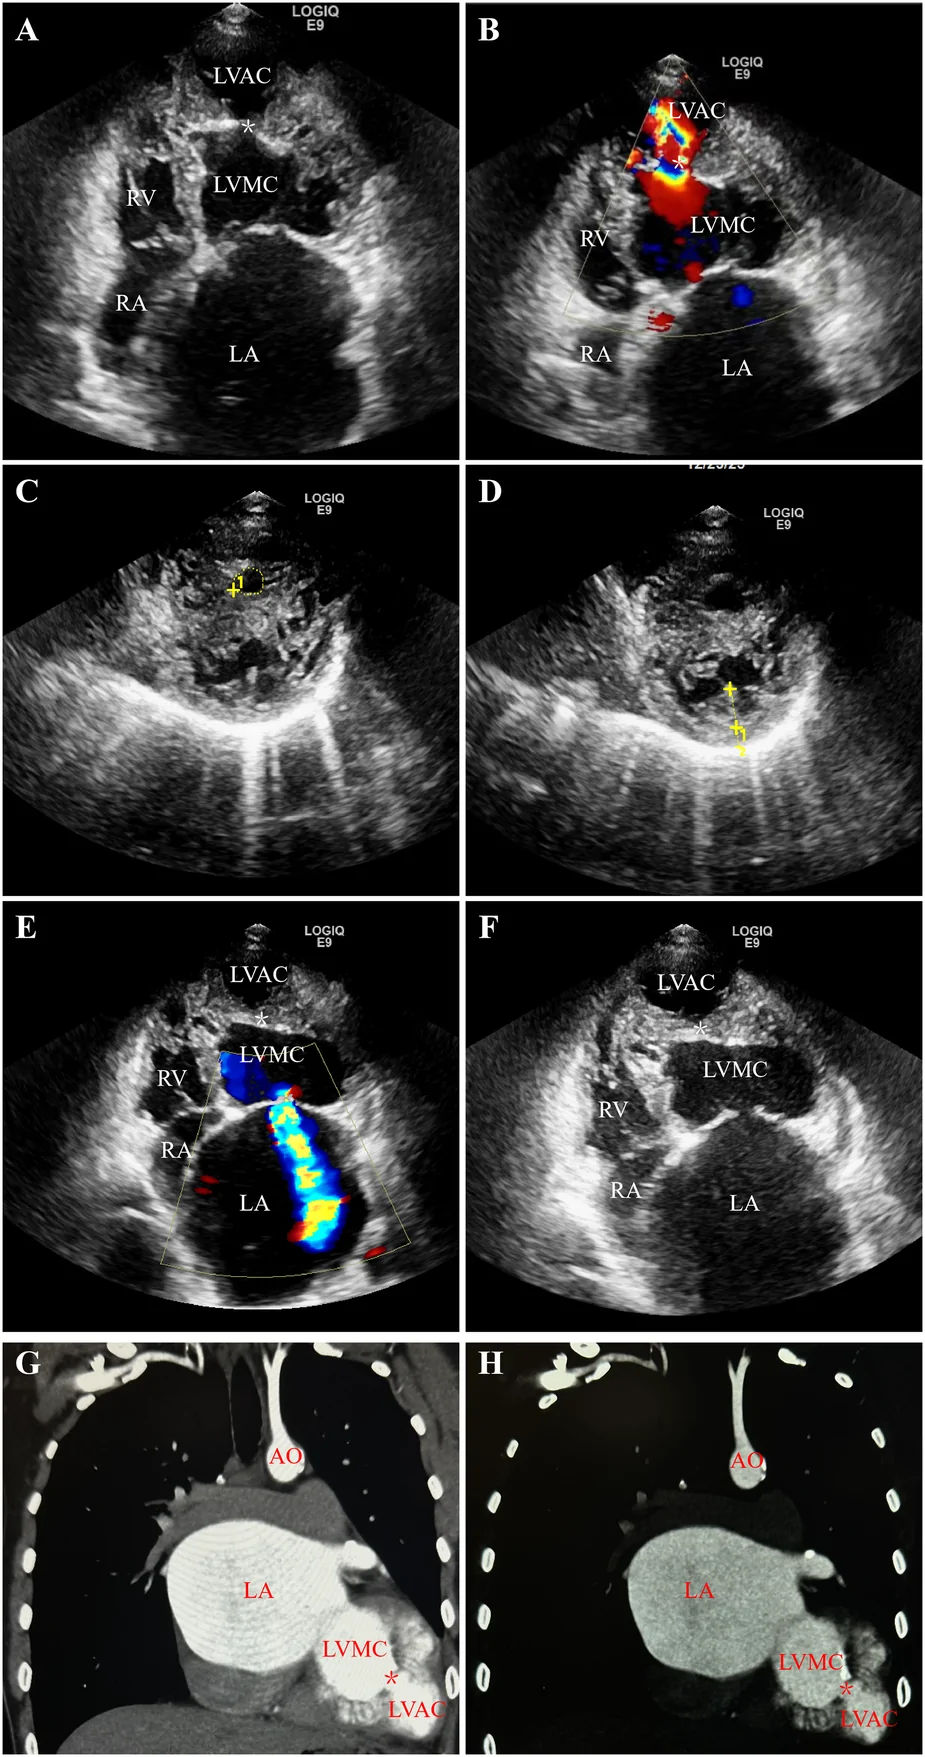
A 50-year-old male with one month of substernal chest tightness and progressive shortness of breath. (A) Echocardiography (Apical four-chamber view): Enlarged left heart is observed. The mid-to-lower segments of the left ventricular wall show thickening and calcification, dividing the left ventricular cavity into two chambers - one near the apex and the other adjacent to the mitral valve. (B) Echocardiography (Four-chamber view, Color Doppler flow imaging): Bidirectional blood flow signals between the two left ventricular chambers are visualized. (C) Parasternal short-axis view, sagittal ultrasound image of the intraventricular septation in the left ventricle. The measurement indicates the area of the communication opening between the two chambers. (D) Parasternal short-axis view. The caliper measurement indicates the thickness of the non-compacted and compacted myocardium of the left ventricular posterior wall. (E) Apical four-chamber view showing mitral regurgitation jet. (F) Apical four-chamber view, long-axis ultrasound image of the intraventricular septation in the left ventricle. (G) Aortic CTA (Arterial phase, original images): Enlarged left heart and double-chambered left ventricle. (H) Aortic CTA (Arterial phase, with adjusted grayscale): Enlarged left heart and double-chambered left ventricle. After grayscale adjustment, focal myocardial thickening with calcification is observed in the mid-portion of the left ventricle. RA, Right atrium; LA, Left atrium; RV, Right ventricle; LV, Left ventricle; LVMC, Left ventricle main cavity; LVAC, Left ventricle accessory cavity; Ao, aorta; *Muscular septum within the mid-to-lower left ventricular cavity.
